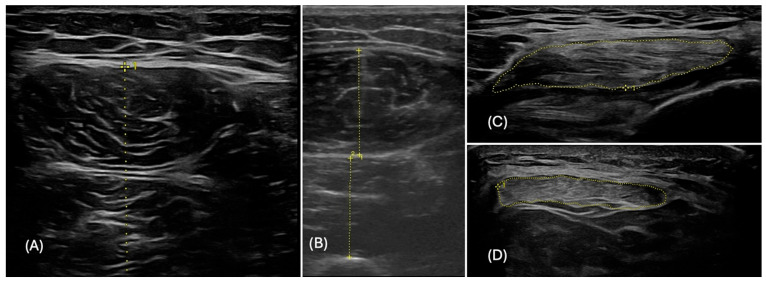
Representative ultrasonographic images obtained during ultrasonographic evaluation. (A,B) Measurement of rectus femoris and vastus intermedius muscle thicknesses. (C) Ultrasonographic appearance and contouring of the quadriceps tendon. (D) Ultrasonographic appearance and contouring of the patellar tendon. The yellow dotted lines indicate the muscle thickness measurements, and the yellow contours indicate the tendon boundaries.
